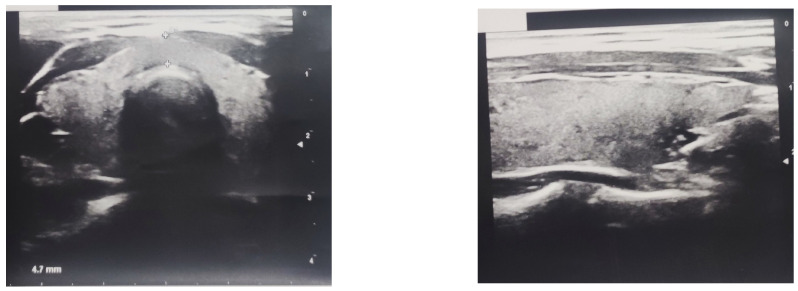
Ultrasound scan of patient’s hypoechogenic, non-homogeneous-structured, normal-sized thyroid gland.
